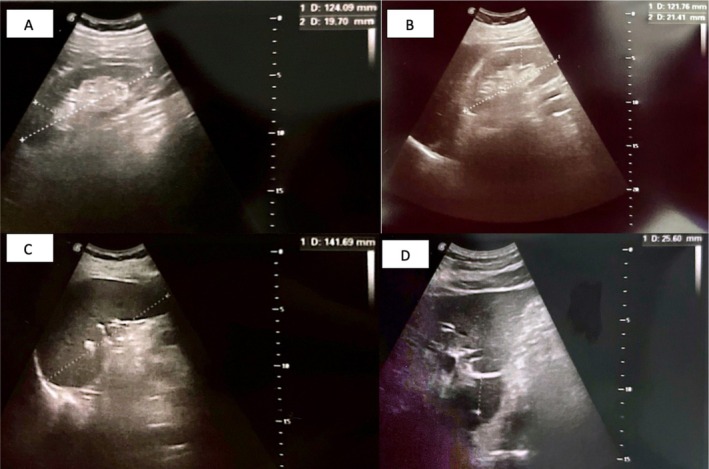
Abdominal ultrasound. (A) Right kidney with a longitudinal axis of 12.4 cm and parenchymal thickness of 2.0 cm, without renal calculi or pelvicalyceal dilatation. (B) Left kidney with a longitudinal axis of 12.2 cm and parenchymal thickness of 2.1 cm, likewise without calculi or pelvicalyceal dilatation. (C) Spleen measuring 14.1 cm in maximal diameter, with homogeneous echotexture. (D) Left hepatic lobe (anteroposterior diameter ≈ 6.0 cm) and caudate lobe (diameter ≈ 2.6 cm), both showing homogeneous echotexture.
